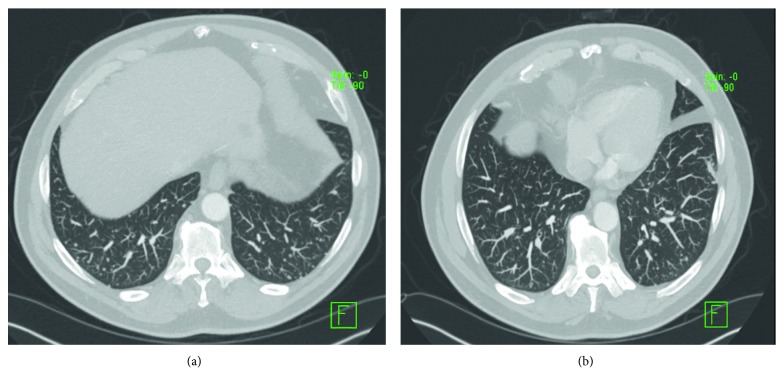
Chest CT showing numerous small lung nodules.
